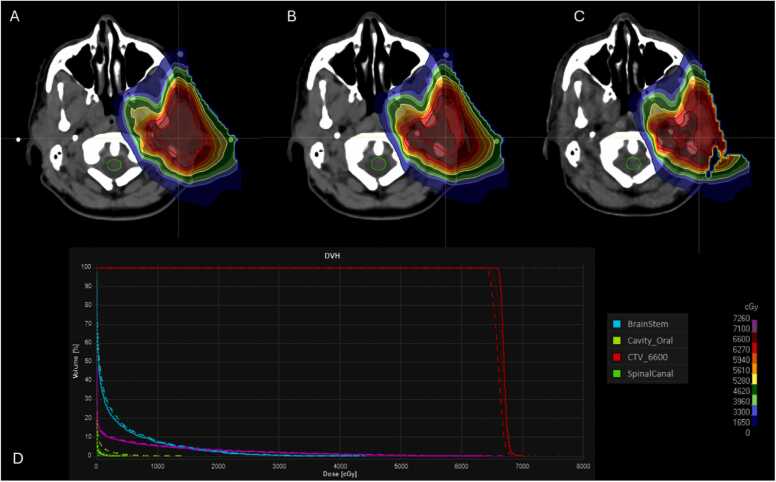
A representative slice of dose distribution comparison from the head-neck cancer patient and among nominal SPArc_-step&shoot_ plan calculation on the initial CT, QA-CT on 11th fx and synCT from CBCT on 11th fx (A) nominal plan on initial CT; (B) nominal plan on QA-CT; (C) nominal plan on synCT; (D) dose-volume histograms (solid line: initial CT; dotted line: QA-CT; and short dashed line: synCT). Abbreviation: CBCT, cone beam computed tomography; QA, quality assurance.
